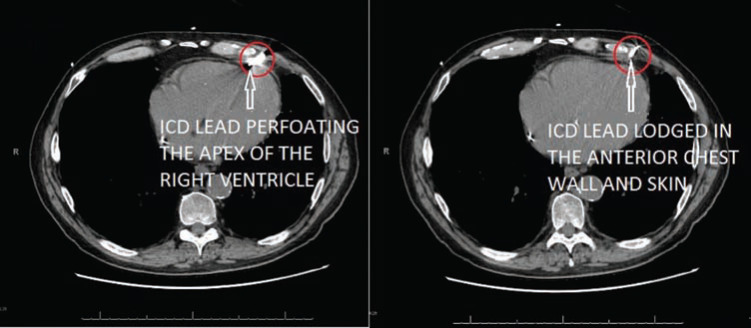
Chest CT showing the location of the lead tip without pericardial effusion, fluid collection, pneumomediastinum, or pneumothorax.
